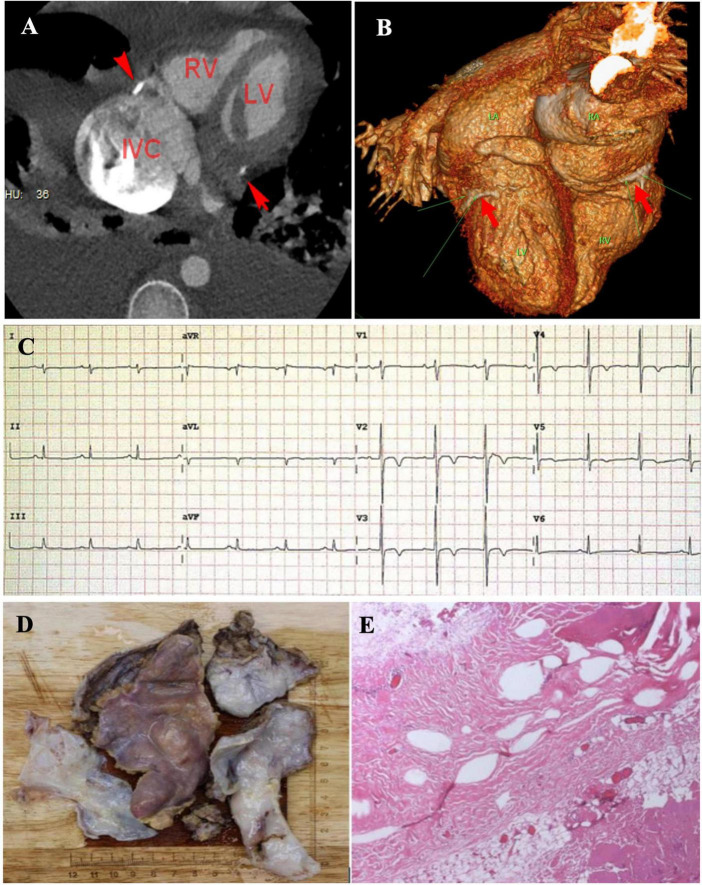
Cardiac computed tomographic angiogram of four-chamber view (A) and volume rendering views (B) showed calcified annular constrictive pericarditis (ACP) trapping both ventricles (red arrows). Spontaneous conversion from atrial fibrillation (AF) to sinus rhythm as the calcified pericardial ring was severed (C). Complete pericardiectomy was performed and revealed a thickened pericardium with calcification embedded in the atrioventricular (AV) groove (D). Pathological examination of the pericardial tissue indicated fibrotic tissue with calcification (E).
